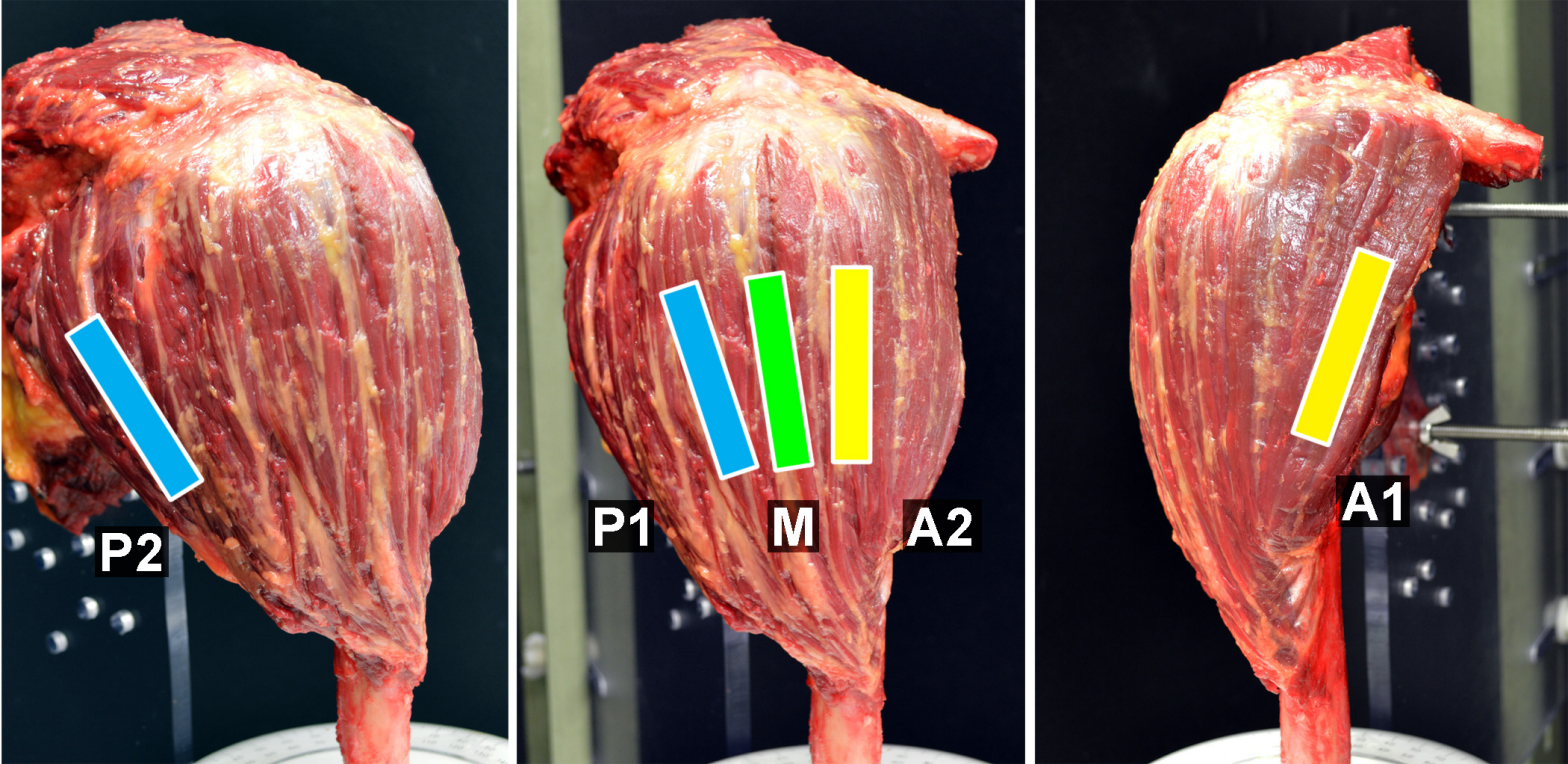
Deltoid muscle anatomy for positioning of ultrasound probe during SWE measurements. SWE was examined percutaneously and the values were obtained from 5 segments; anterior (A1, A2), middle (M), and posterior (P1, P2).
